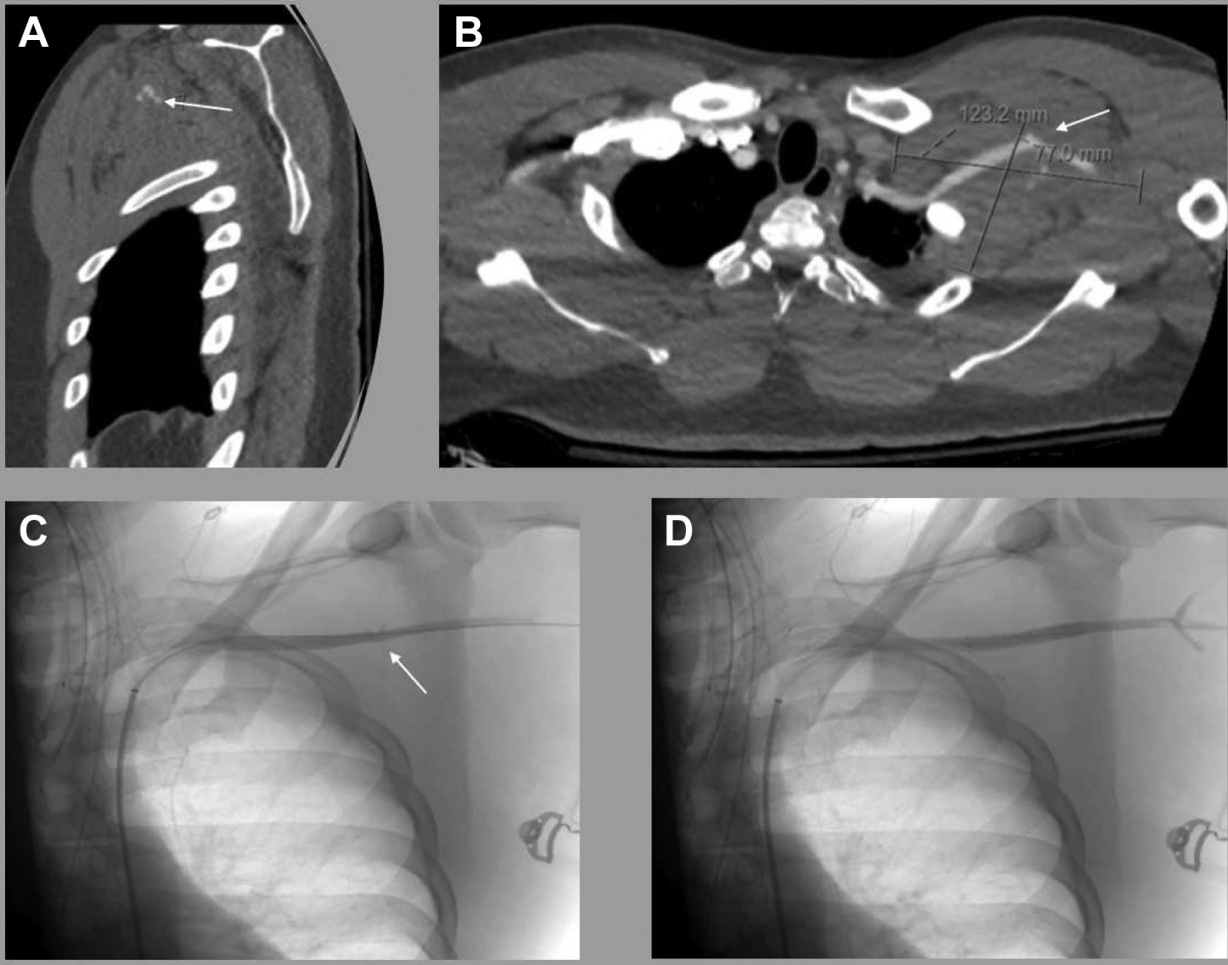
Preoperative and completion imaging findings for patient 4 with left subclavian artery transection. A, B, Computed tomography angiograms demonstrating 7.7 × 12.3-cm retroclavicular hematoma with areas of active arterial extravasation (*arrows*) within the hematoma. C, Intraoperative digital subtraction angiogram with femoral sheath access. Note arterial blush of the left subclavian artery (*arrow*). D, Completion digital subtraction angiogram after stent placement with no evidence of arterial extravasation.
